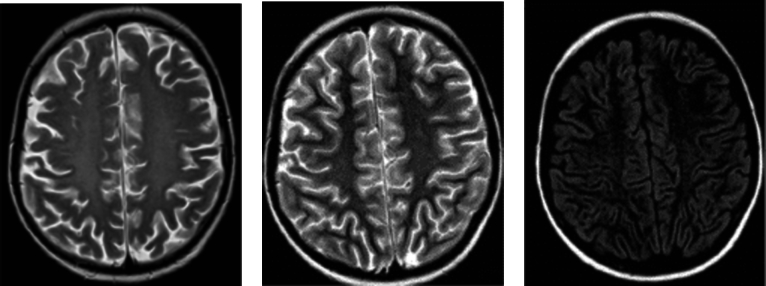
Types of MR images in the Kaggle dataset: a T1-Weighted type image is shown on the left, a T2-Weighted type image in the center, and a Flair-type image on the right.
